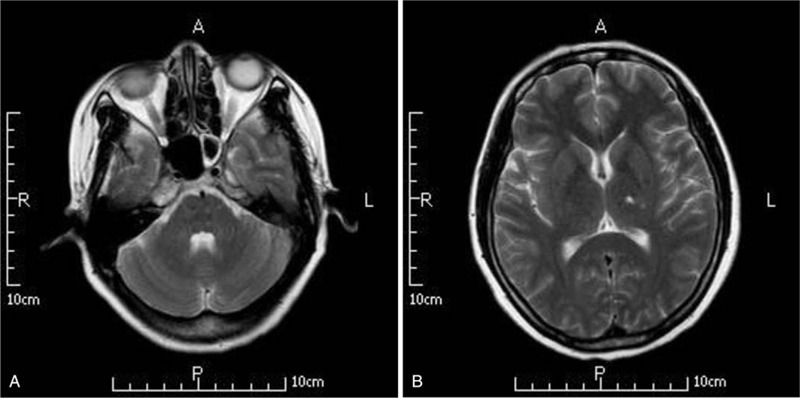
(A and B) Cerebrospinal fluid magnetic resonance imaging (MRI) of the brain showed in both sides of the cerebral cortex, the left basal ganglia, the left thalamus, the middle brain, the pontine, the right cerebellar hemisphere, there were many abnormal signals. T1WI showed low signal, T2WI, T2-FLAIR showed high signal, DWI showed high signal, the enhanced scan was ring strengthening, and no obvious abnormal enhancement was found in the meninges.
